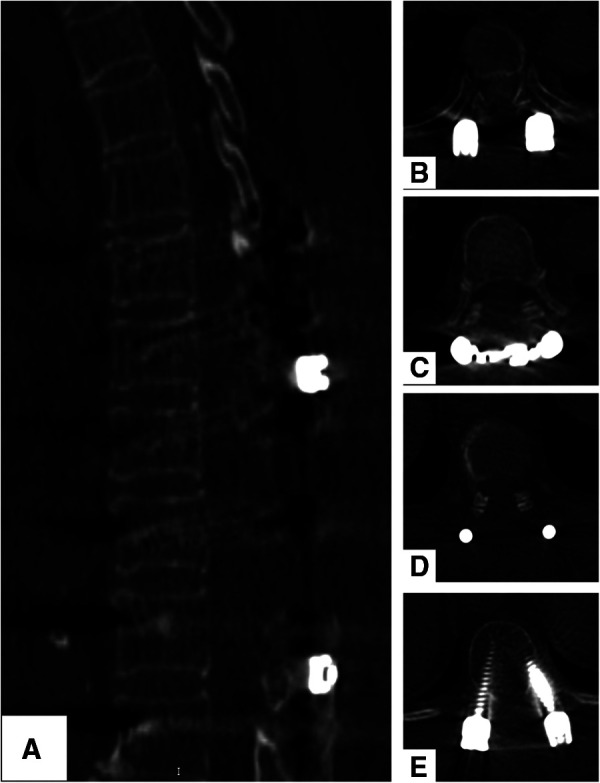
(A–E) CT scans show a satisfactory decompression of the spinal cord, and no recurrence spinal meningioma was found after 2 years.
